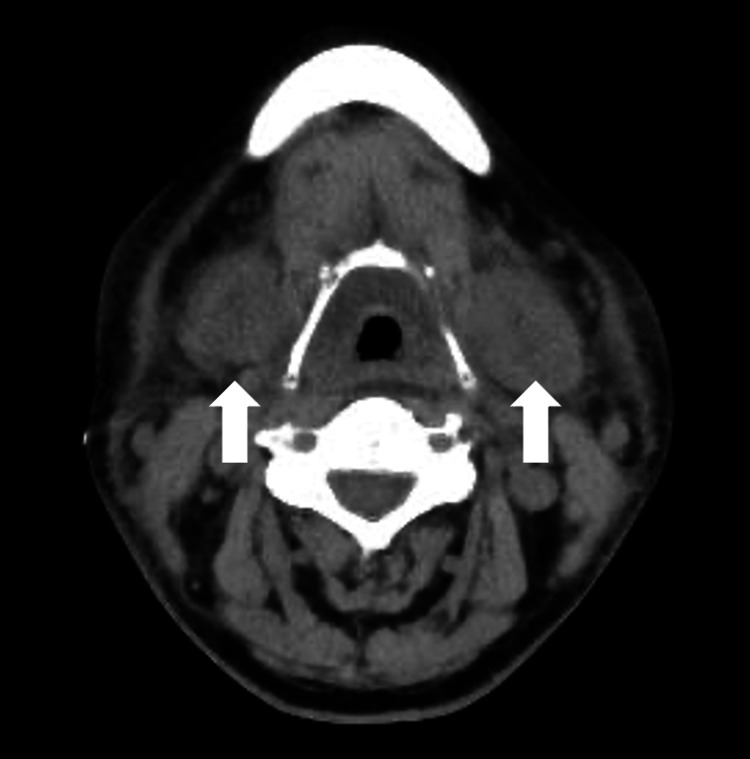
CT findings at the onset of local CRS, showing bilateral submandibular gland swelling. CT: computed tomography; CRS: cytokine release syndrome.
